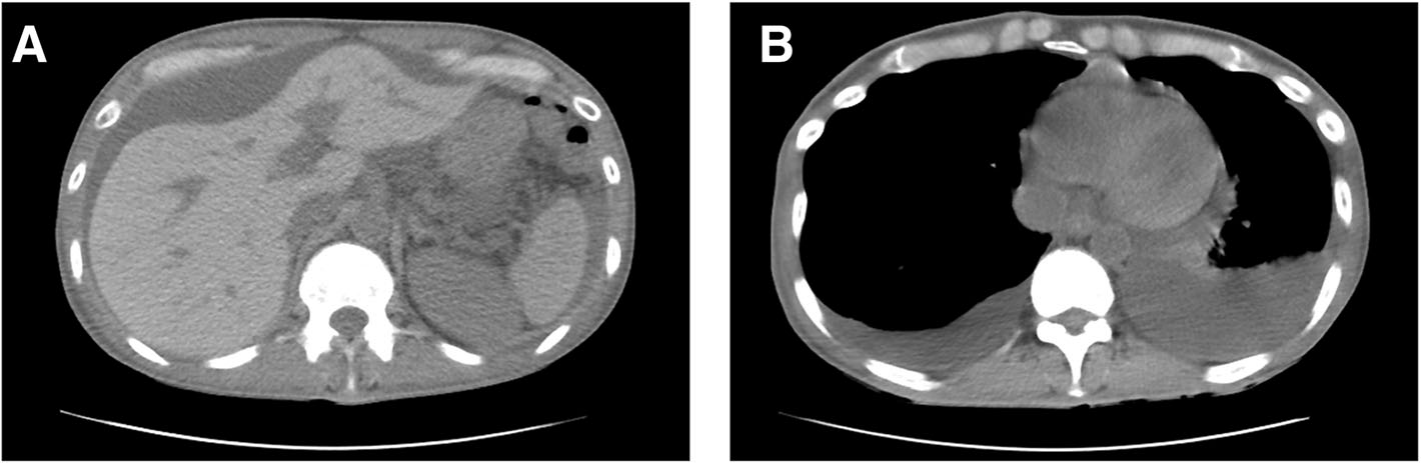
Computed tomography findings. Computed tomography findings at the time of development of persistent ascites (a) and pleural effusion (b)
